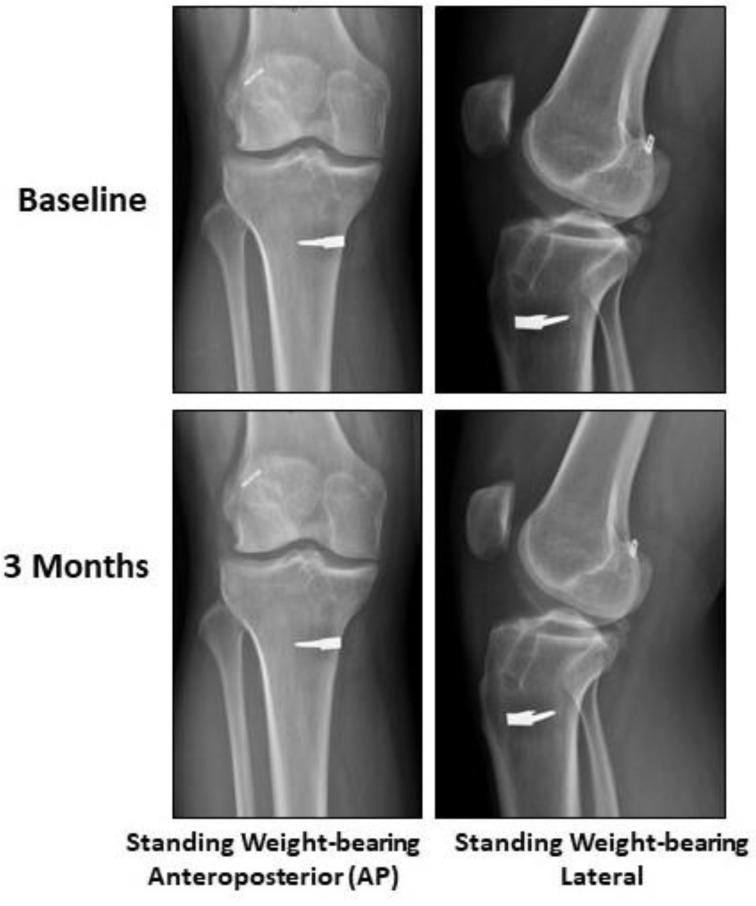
Plain Radiography. No significant difference nor progression in OA via X-Rays was observed.
